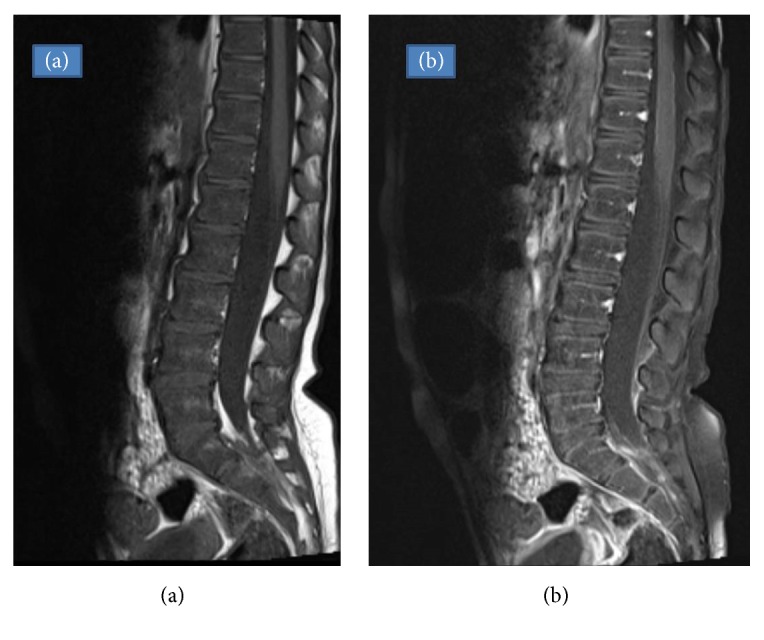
Sagittal T1-weighted image (a) and sagittal postcontrast T1-weighted image with fat suppression (b) show a significant tumor mass volume reduction after treatment.
